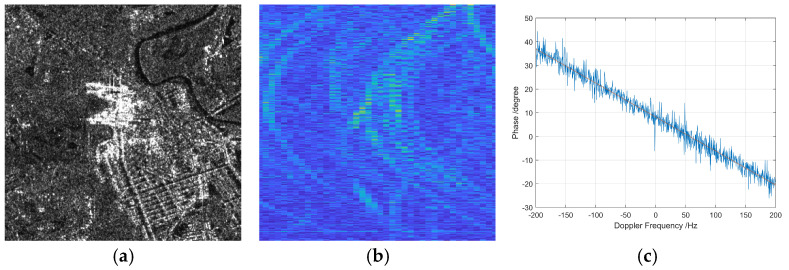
Multi-channel echo range migration curve of power transmission tower. (a) Image slice amplitude. (b) Range migration curve after range compression in time-domain. (c) Channel phase error varying with Doppler frequency.
